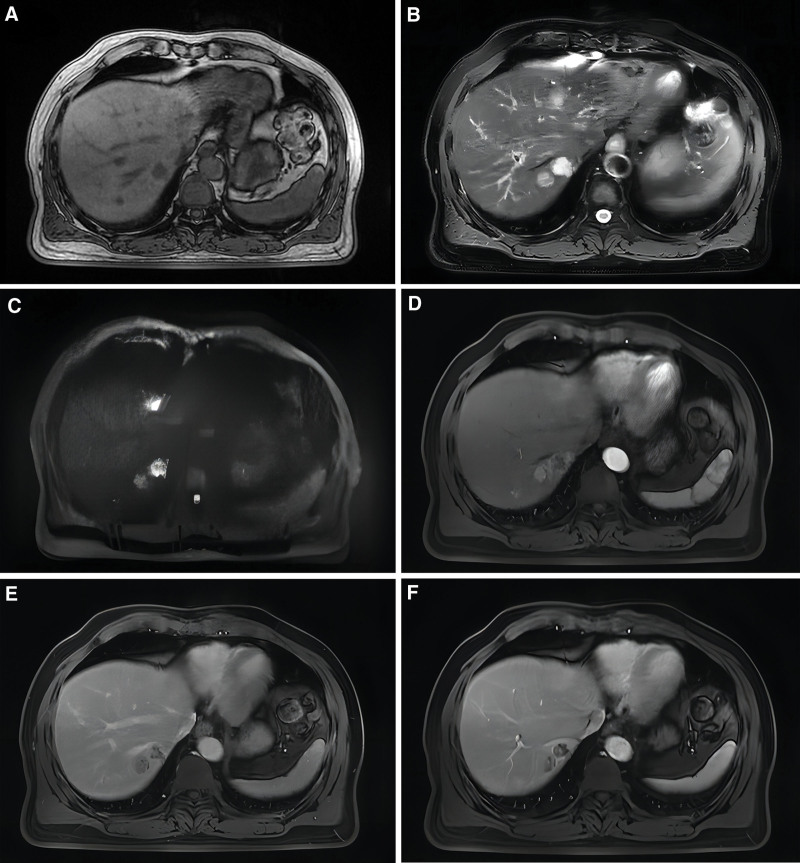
Abdominal MR image before surgery. (A) Lesion shows a mild low signal on T1WI; (B) soft downward motion on T2WI; (C) heterogeneous high signal on DWI sequence; (D) patchy enhancement is seen around the lesion in the arterial phase; (E) low signal in the delayed phase; (F) everyday movement in the portal phase.
